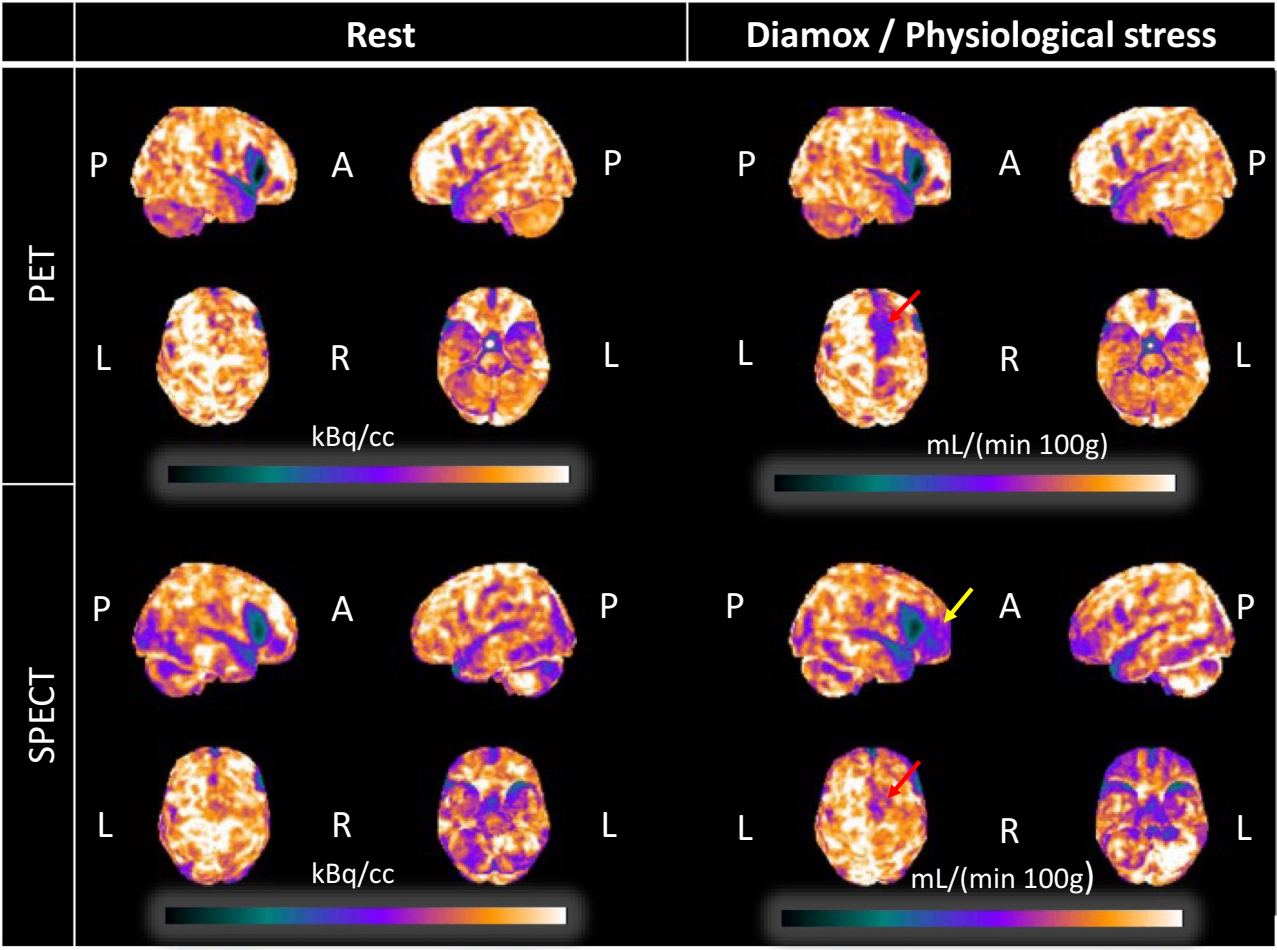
Surface projections of the CBF and SPECT (kBq/cc) and PET (mL/(min∙100 g)) scan during rest and physiological stress or Diamox. The images have been visually scaled to approximate the global mean, facilitating comparison. The yellow arrow indicates an area affected only on the SPECT scan, while the red arrows show a reduction in the mesial frontal lobe, most notably observed on the PET scan. P: posterior, A: anterior, L: left, R: right
